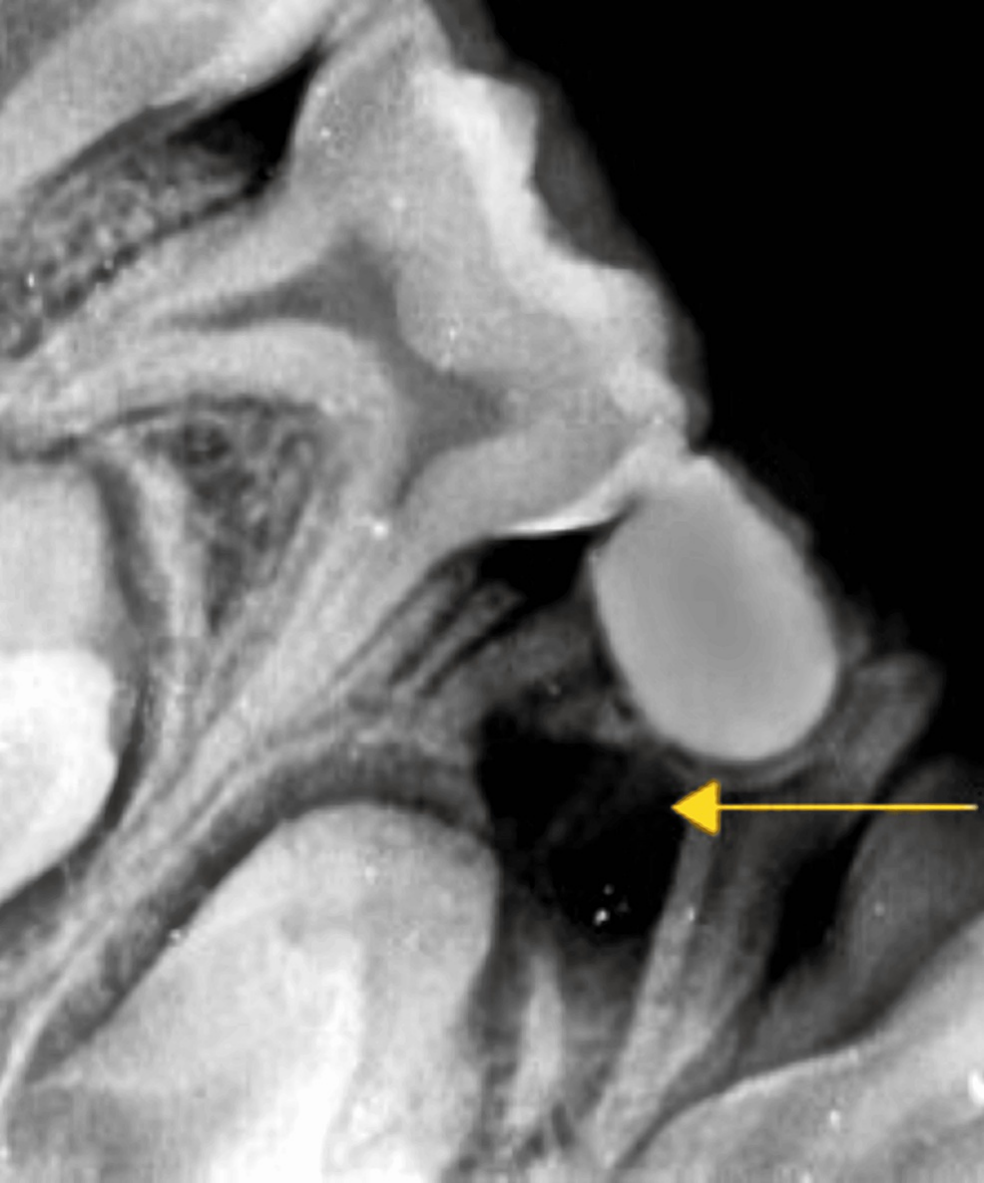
Depiction of the radiograph of RE of the left deciduous mandibular first molar at nine-month follow-up RE: Radix entomolaris
